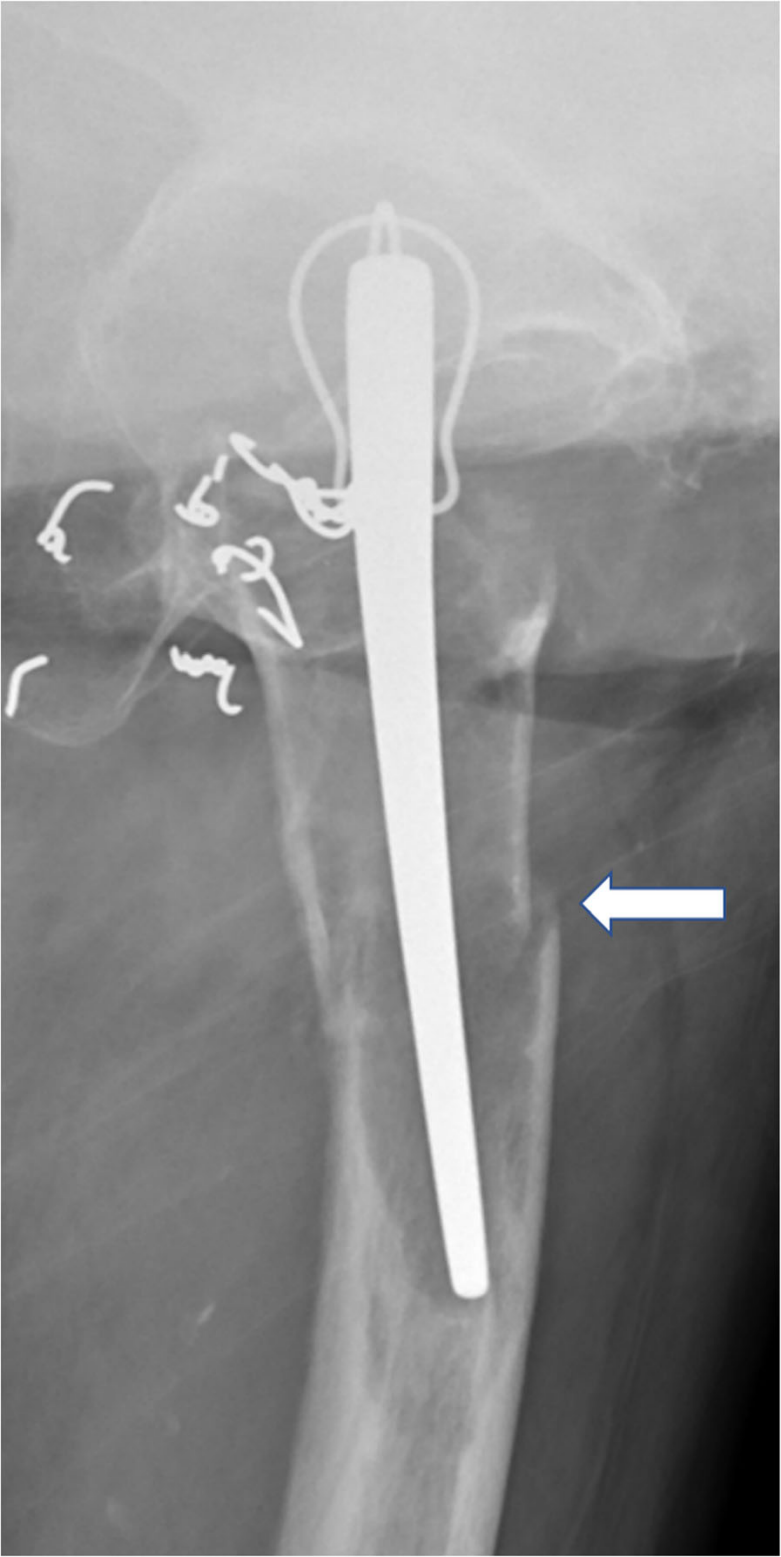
A plain lateral radiograph of the left hip at the patient’s first visit showing periprosthetic fracture of the left femur (arrow)
